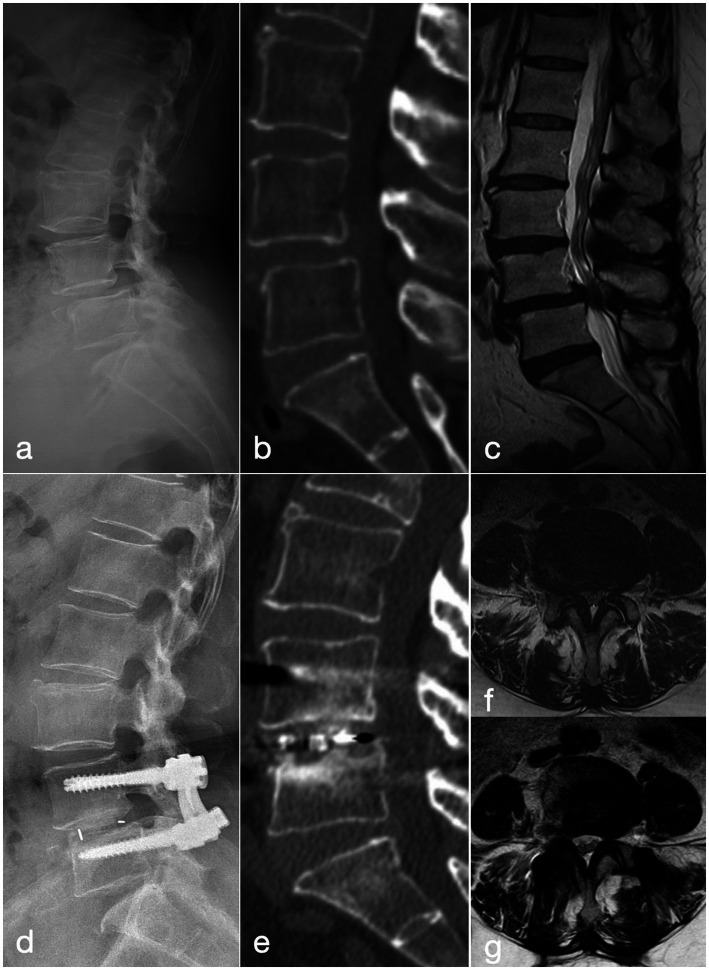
A 57-year-old woman with recurrent low back pain and lower limb intermittent claudication of 9 months’ duration, was treated with the MIS-TLIF procedure. (a–c) Pre-operative lateral radiograph, CT and MRI show grade-I anterior spondylolisthesis of L4. (d) Post-operative lateral radiograph demonstrates well-positioned instrumentation and interbody cage with good reduction of the slip. (e) CT at 2 years confirms solid interbody fusion at L4/5. (f) Pre-operative MRI reveals severe L4/5 central canal stenosis. (g) MRI at 2 years shows increased canal volume and patent cerebrospinal fluid signal.
